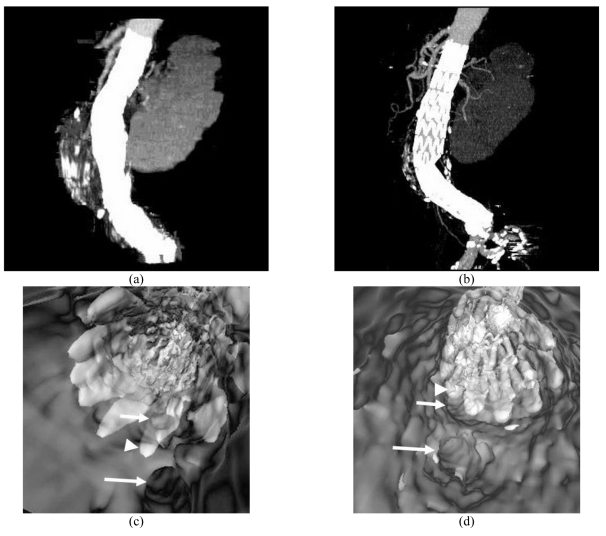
An 81-year-old woman with an abdominal aortic aneurysm was treated with a suprarenal stent graft (A) and followed-up at 36 months. A distal stent graft migration of 10.2 mm was noted in the most recent CT MIP image (B). VE (C) at 1 week of stent graft implantation showed that a stent wire crossed the SMA ostium (short arrow), but at 36 months (D), the suprarenal stent wires had shifted (short arrow). Long arrow: celiac axis, arrowhead: suprarenal stent wires.
